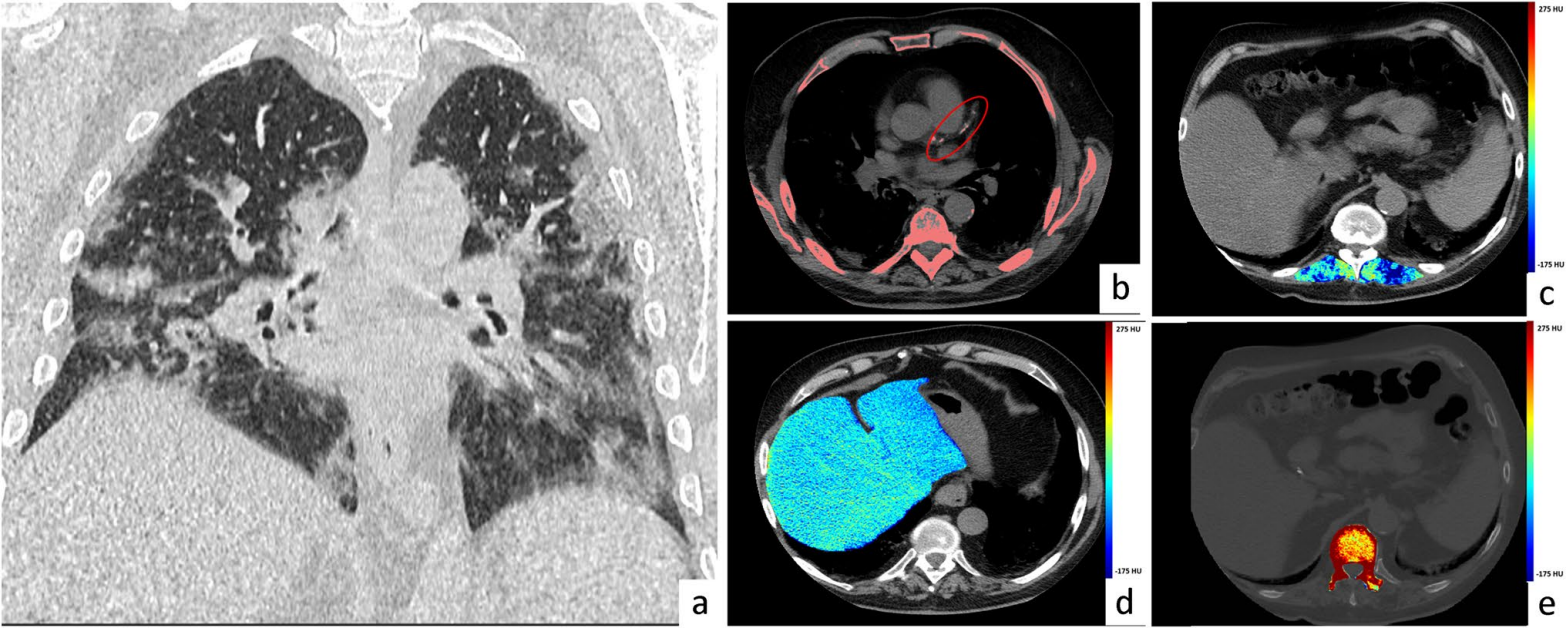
Exemplifying patient treated with non-invasive ventilation. Male patient,75 y.o., with a clinical history of hypertension and diabetes. At admission to the hospital SaO2 in ambient air was 80%. Main laboratory data were: WBC 6200/mm3, C-Reactive Protein 2.64 mg/dL. CT scan was performed after two days after admission, revealing a moderate involvement of the lung parenchyma (Pneumonia score 3, a), a low coronary artery calcium score (Agatston score 46, b), reduced liver attenuation, indicating the presence of steatosis (mean density of right and left lobe 25.5 HU, d), presence of myosteatosis (mean attenuation of paravertebral muscles 23 HU, c), and osteoporosis (bone density 126 HU, e). The patient was admitted to the hospital, and during the hospitalization needed non-invasive ventilation. He was discharged after 34 days
